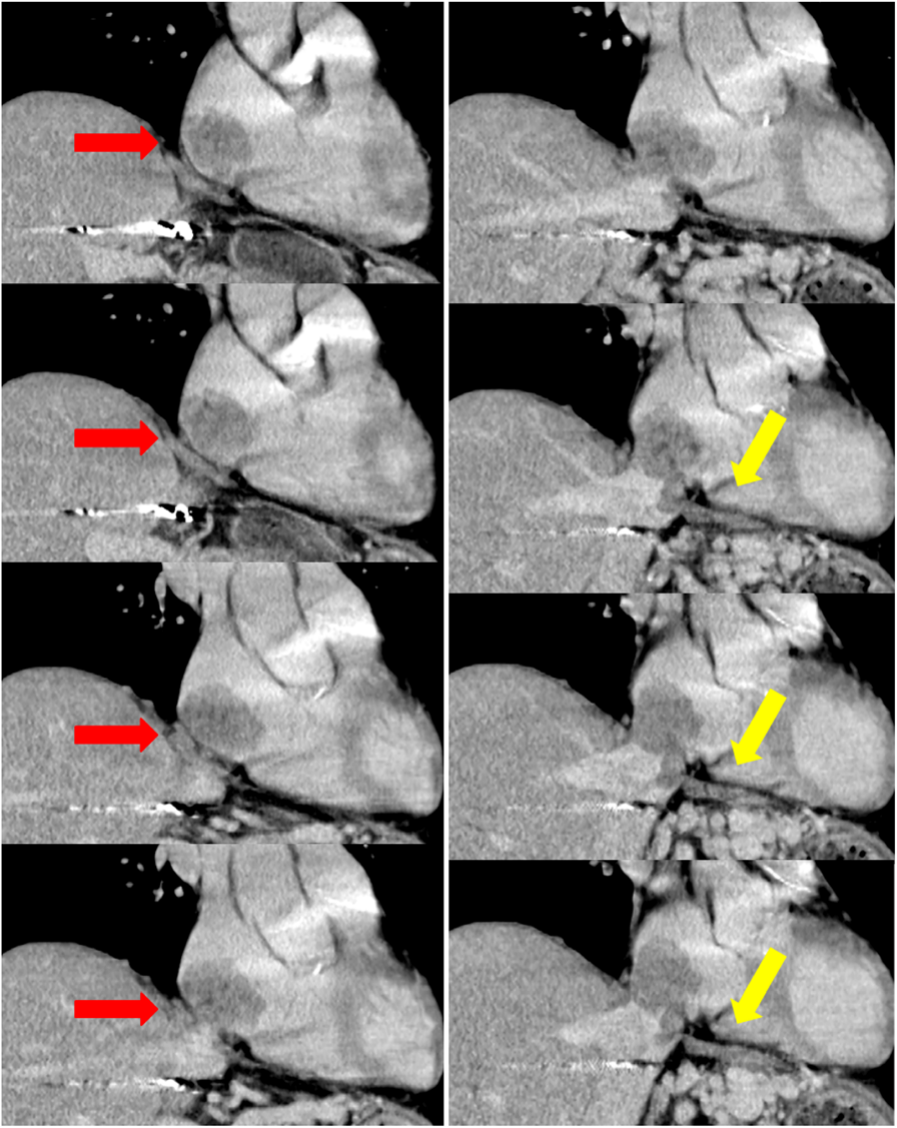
Dynamic-enhanced computed tomography imaging of case 1 (reconstruction for the coronal axis). A tumor thrombus was detected in both the inferior vena cava (IVC) and right atrium (RA) (red arrow), with extension to the left phrenic vein (yellow arrow)
